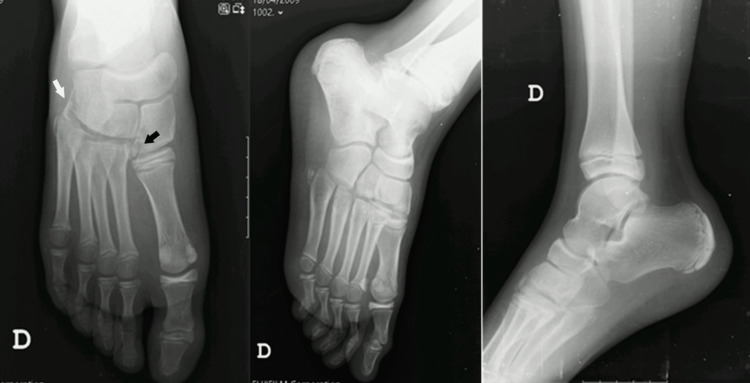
Initial X-rays of the right foot and ankle. Black arrow represents the fracture of the lateral aspect of the medial cuneiform (“fleck sign”); White arrow represents the fracture of the cuboid and subluxation of the M2-M5 tarsal-metatarsal joints.
